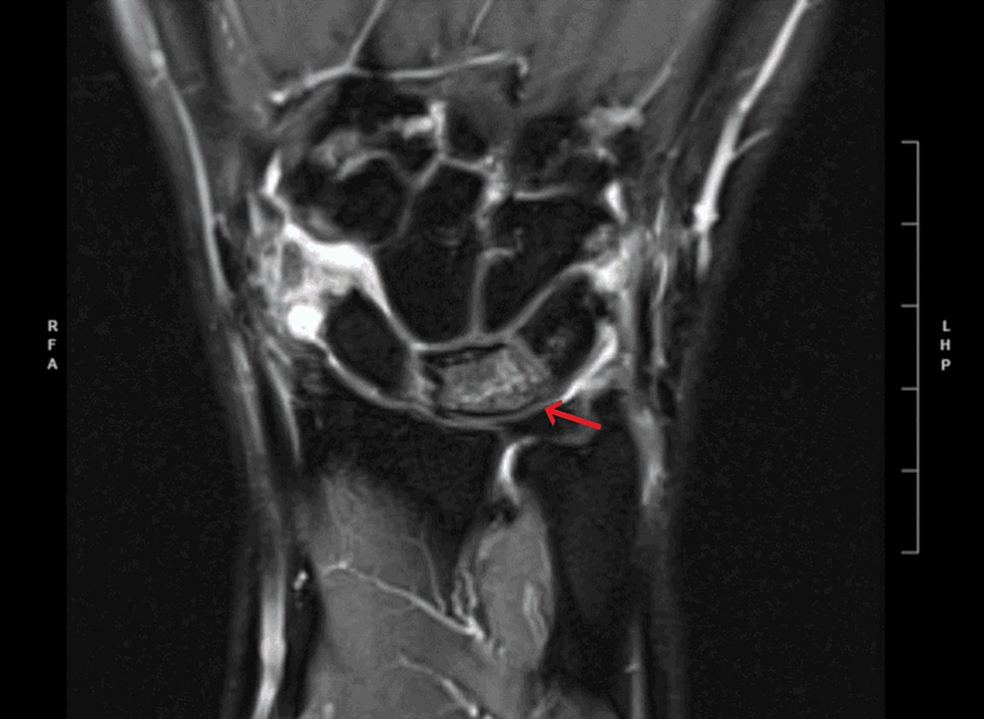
T2 Coronal MRI The arrow corresponds to diffuse increased signal intensity in the lunate bone compared to the T1 series, which may be representative of avascular necrosis. Image #11/19; spin echo (SE): 6001
